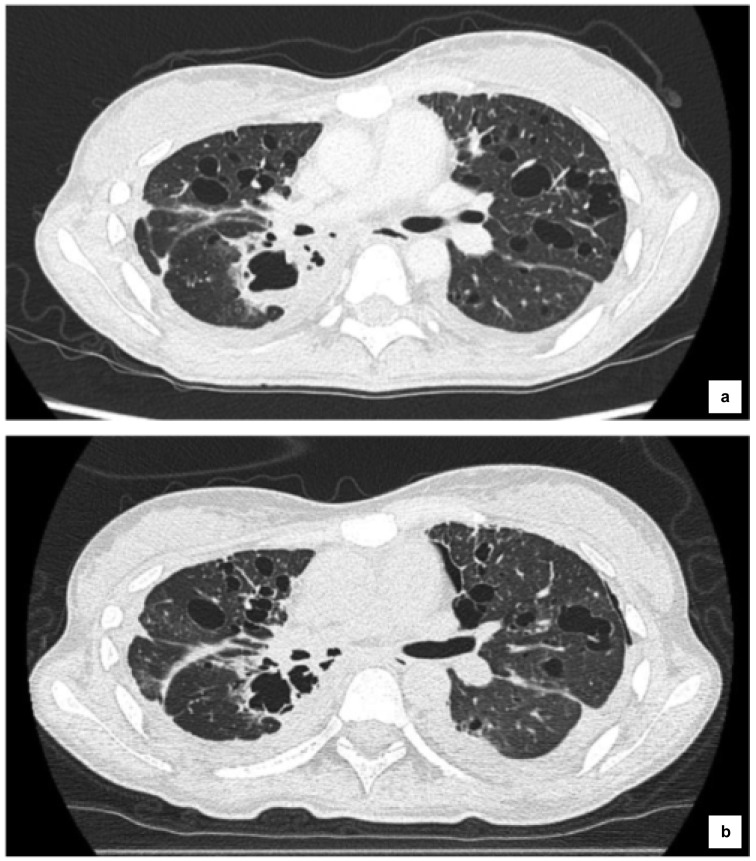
CT images after administration of osimertinib. Cavitation and decrease in size of the primary lesion were observed after 43 (a) and 81 days (b).
